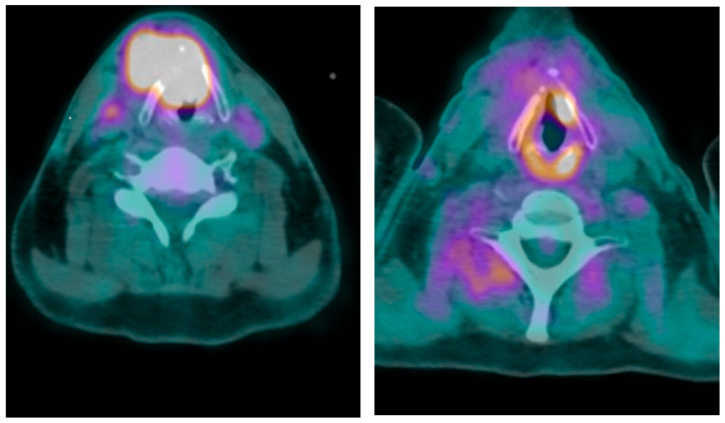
TEP-CT images showing the hypermetabolic activity of the tumor before treatment (left) and the metabolic response after treatment (right).
